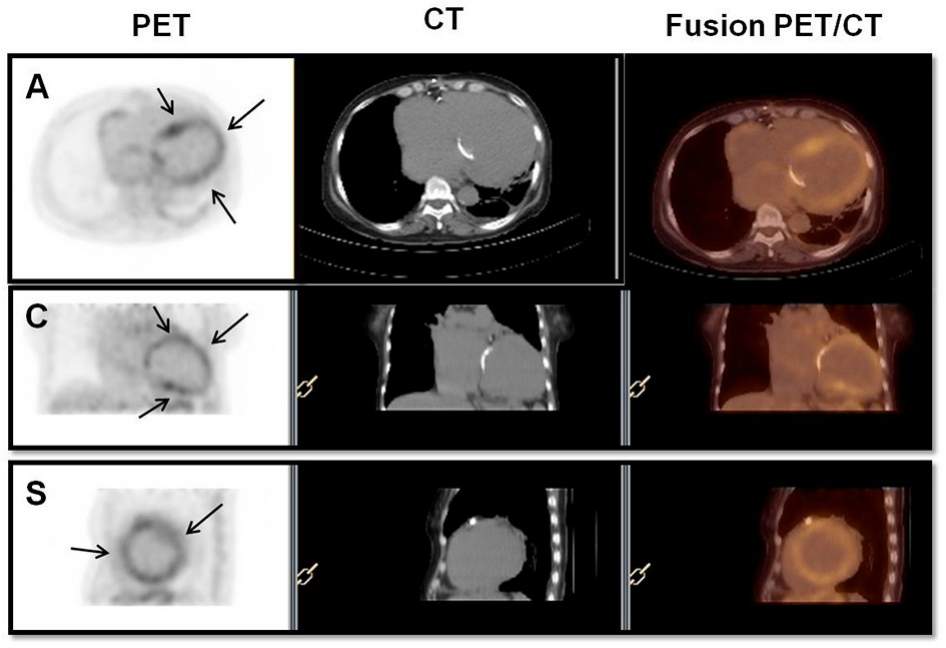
Positive ^18^F-FDG PET/CT scan. Fluorine-18-fluorodeoxyglucose positron emission tomography (18F-FDG PET/CT) (PET, CT, and fusion PET/CT) showing diffuse FDG uptake in the LV myocardium (arrows), indicating active inflammation. The intensity of uptake was discrete, and SUVmax was 3.3. A, axial; C, coronal; S, sagittal; and SUV, standardized uptake value.
